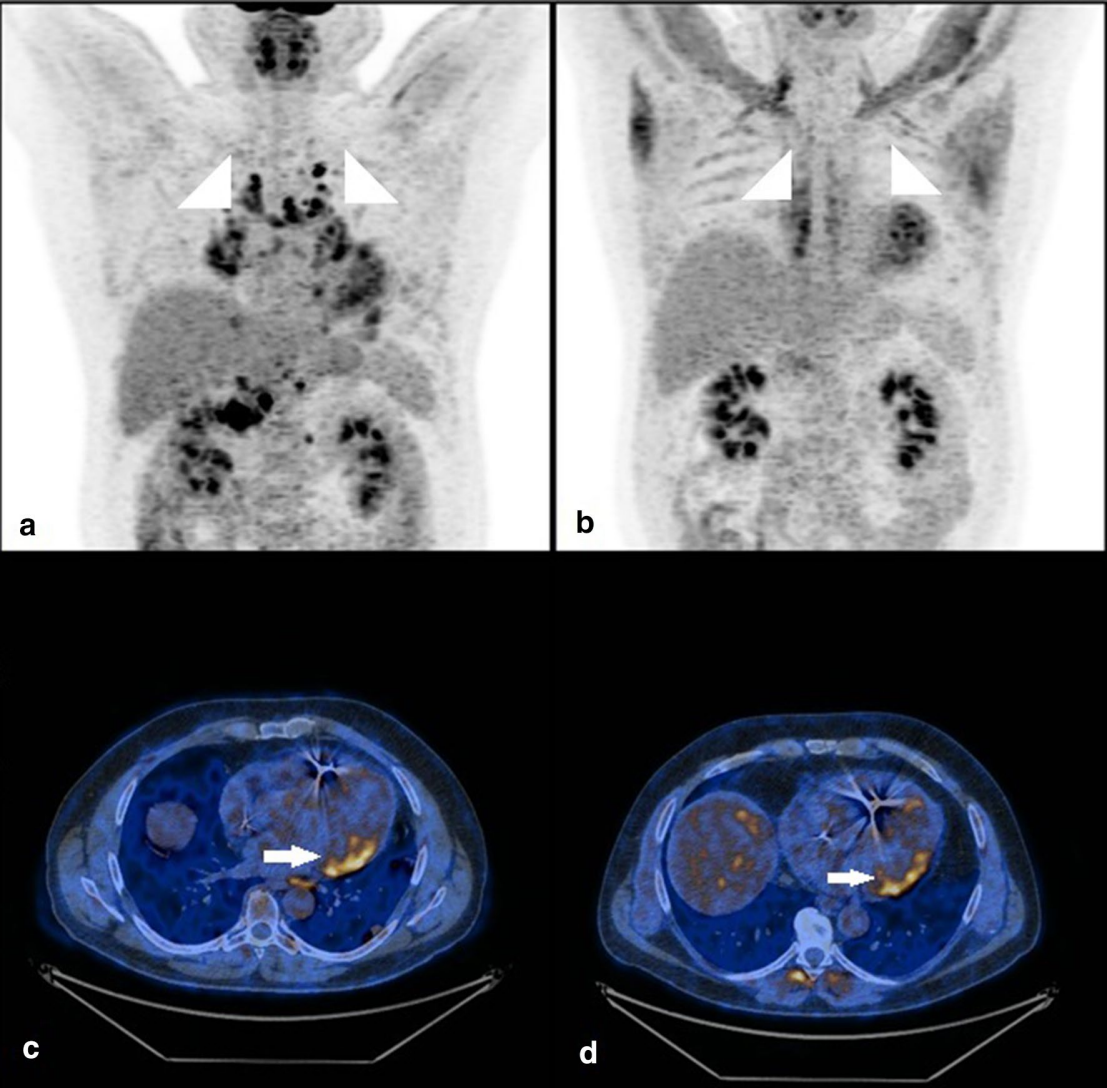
A 54-year-old patient with sarcoidosis. Primary scan (a maximum intensity projection (MIP) and c axial) revealed an increased uptake foci in the inferior lateral segments of the left ventricle (concordant with prior magnetic resonance imaging findings) and active mediastinal lymph nodes. The follow-up scan (b, d) after 4 months of steroid treatment shows good response in lymph nodes, but no significant changes in the heart
